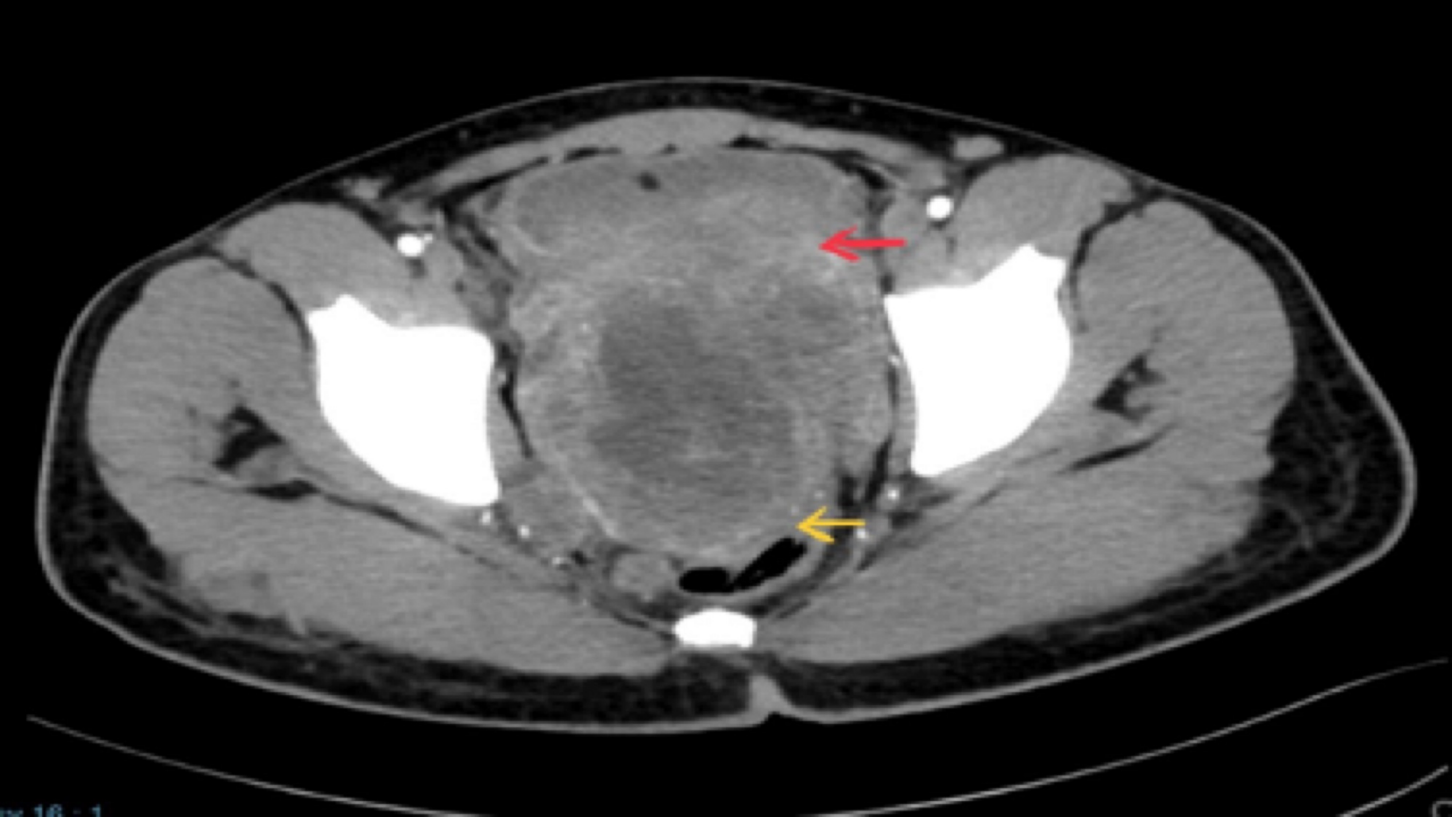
CECT showing the tumor infiltrating the rectum (yellow arrow) posteriorly and bladder (red arrow) anteriorly CECT - contrast-enhanced computed tomography
